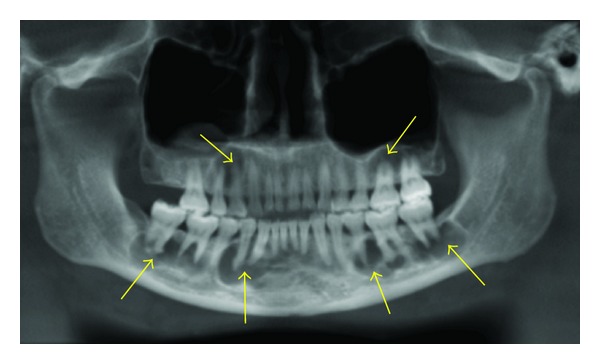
Florid cemento-osseous dysplasia seen as mixed predominantly radiolucent lesions seen in the periapical regions of most of the teeth in the maxillae and the mandible on this panoramic reconstruction image.
